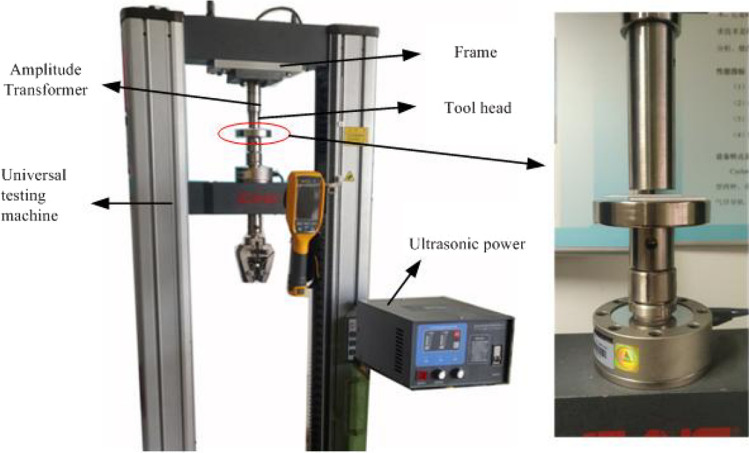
Ultrasonic-assisted riveting test system.
